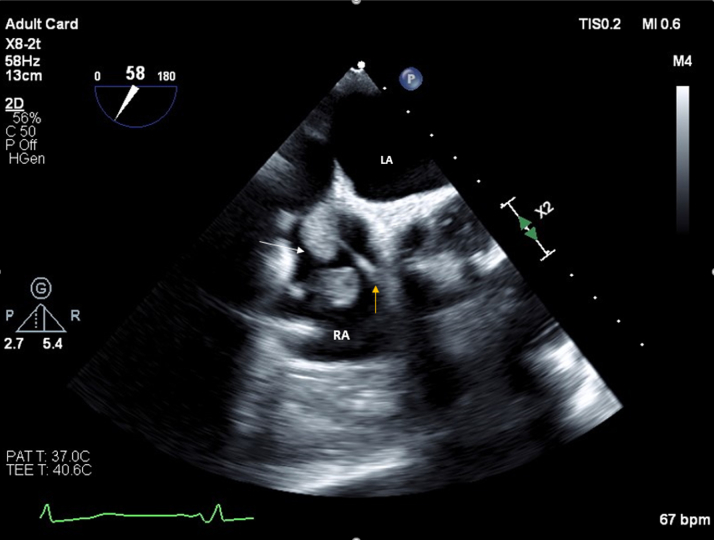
Transesophageal Echocardiography of Echodense Mass Transesophageal echocardiography revealed that the echodensity suspicious for thrombus/vegetation (white arrow) was attached to the right atrial lead (yellow arrow) and tricuspid annulus. LA = left atrium; RA = right atrium.
